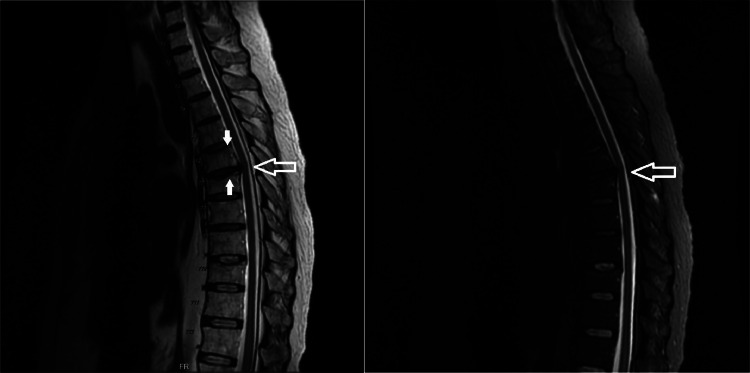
Key images from the MRI of the thoracic spine, with focus placed on the pathological fractures of T6 and T7 (shaded arrows) and degenerative disc disease at the same spinal level causing mild central canal stenosis (nonshaded arrows).
